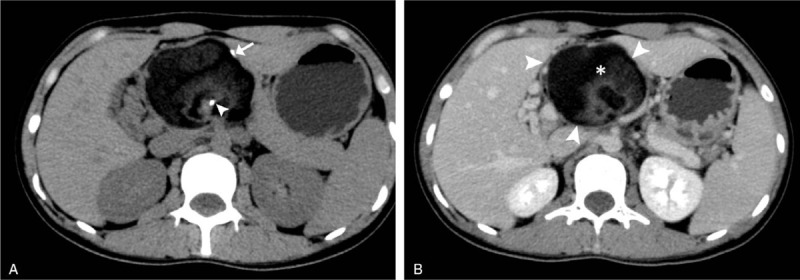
(A) Plain CT showing partially calcified capsule (arrow) with calcification within the mass (arrowhead). (B) Contrast-enhanced CT showing a large heterogeneous mass with slight peripheral enhancement (arrowheads) in the head of the pancreas with a large inhomogeneous area (asterisk).
